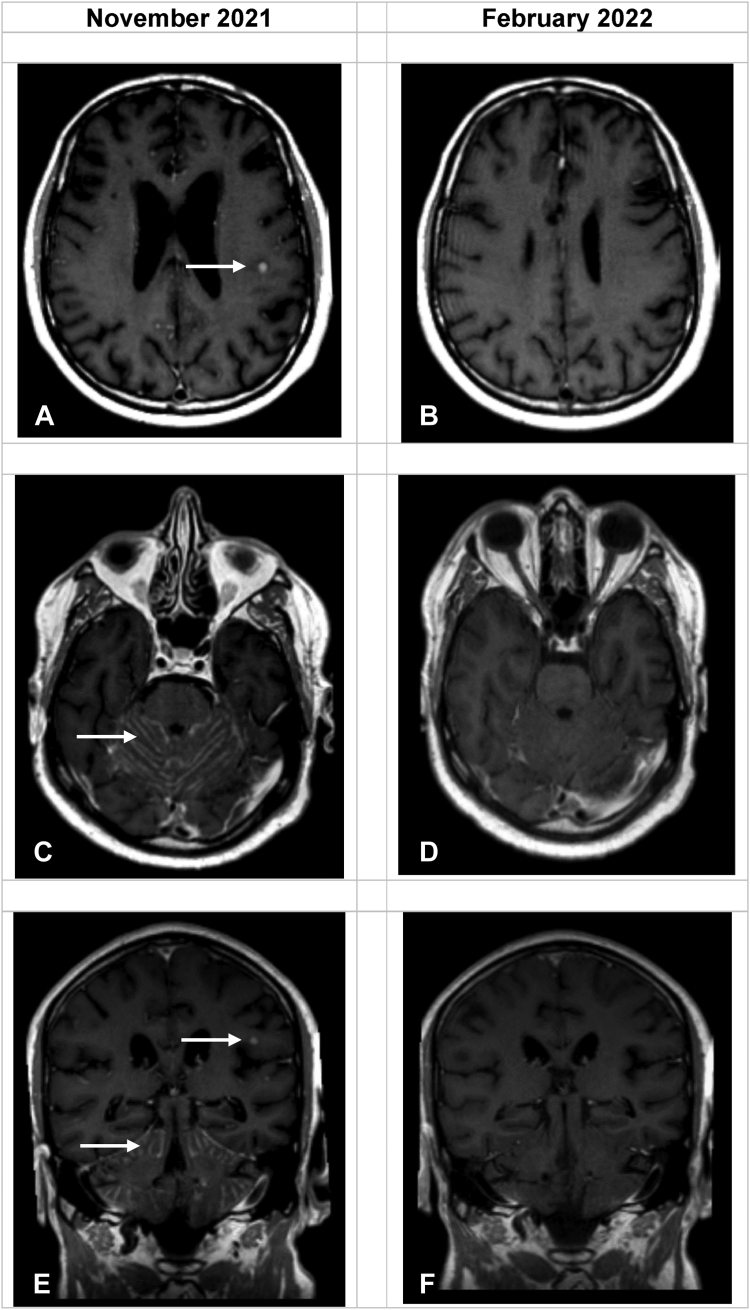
Serial magnetic resonance imaging of the brain. (*A*, *B*) Postcontrast axial T1 sequences at the level of the inferior frontal gyrus. (*C*, *D*) Postcontrast axial T1 sequences at the level of the superior cerebellum. (*E*, *F*) Postcontrast coronal T1 sequences at the level of the occipital horns of the lateral ventricles. The enhancing intra-axial metastasis within the left inferior frontal gyrus and diffuse enhancing leptomeningeal disease of the cerebellum (arrows) have disappeared.
